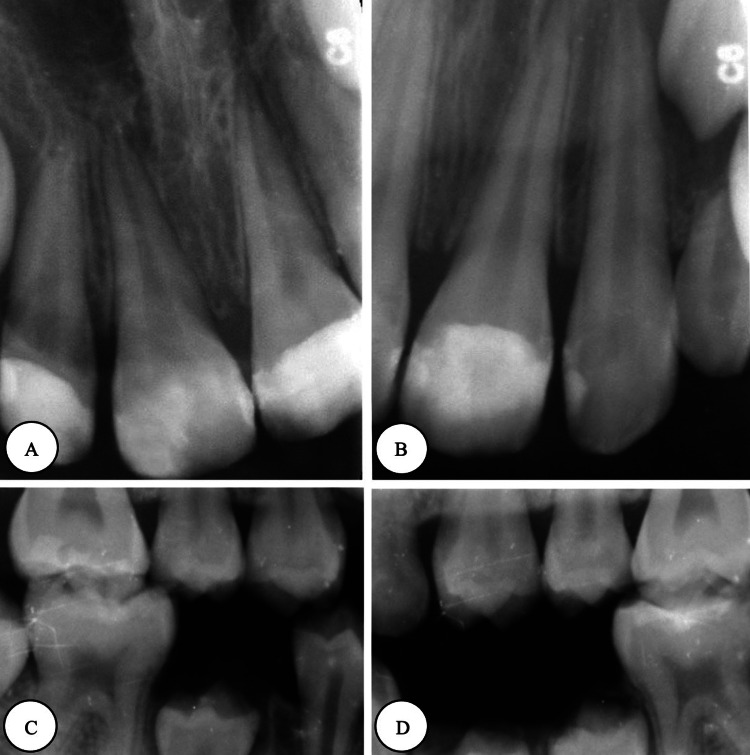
Postoperative radiographs (A) Periapical right-shifted radiograph. (B) Periapical left-shifted radiograph. (C) Right-side bitewing. (D) Left-side bitewing
